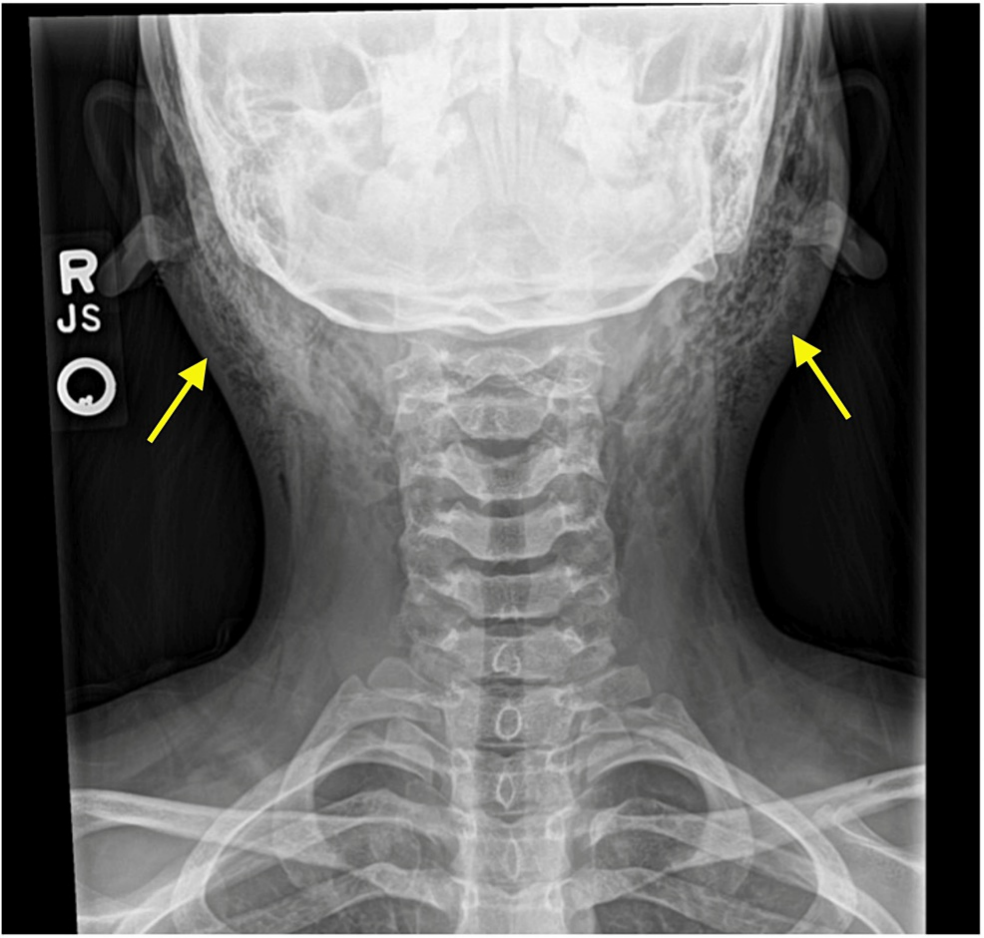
Soft tissue neck X-ray exhibiting subcutaneous emphysema (noted with arrows)
